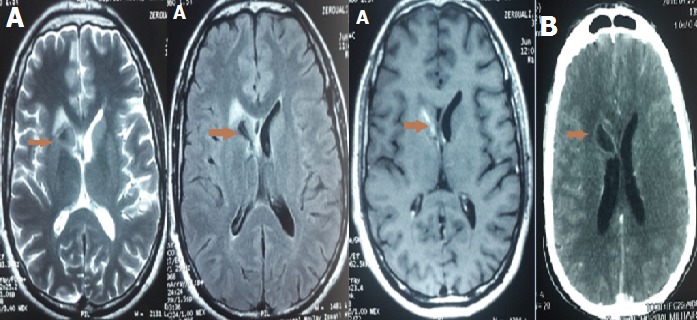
A): IRM cérébrale, en coupe axiale T2, FLAIR et T1 avec injection, montrant un tuberculome cérébrale du noyau caudé; B): TDM cérébrale avec injection, en coupe axiale, montrant un tuberculome du noyau caudé
